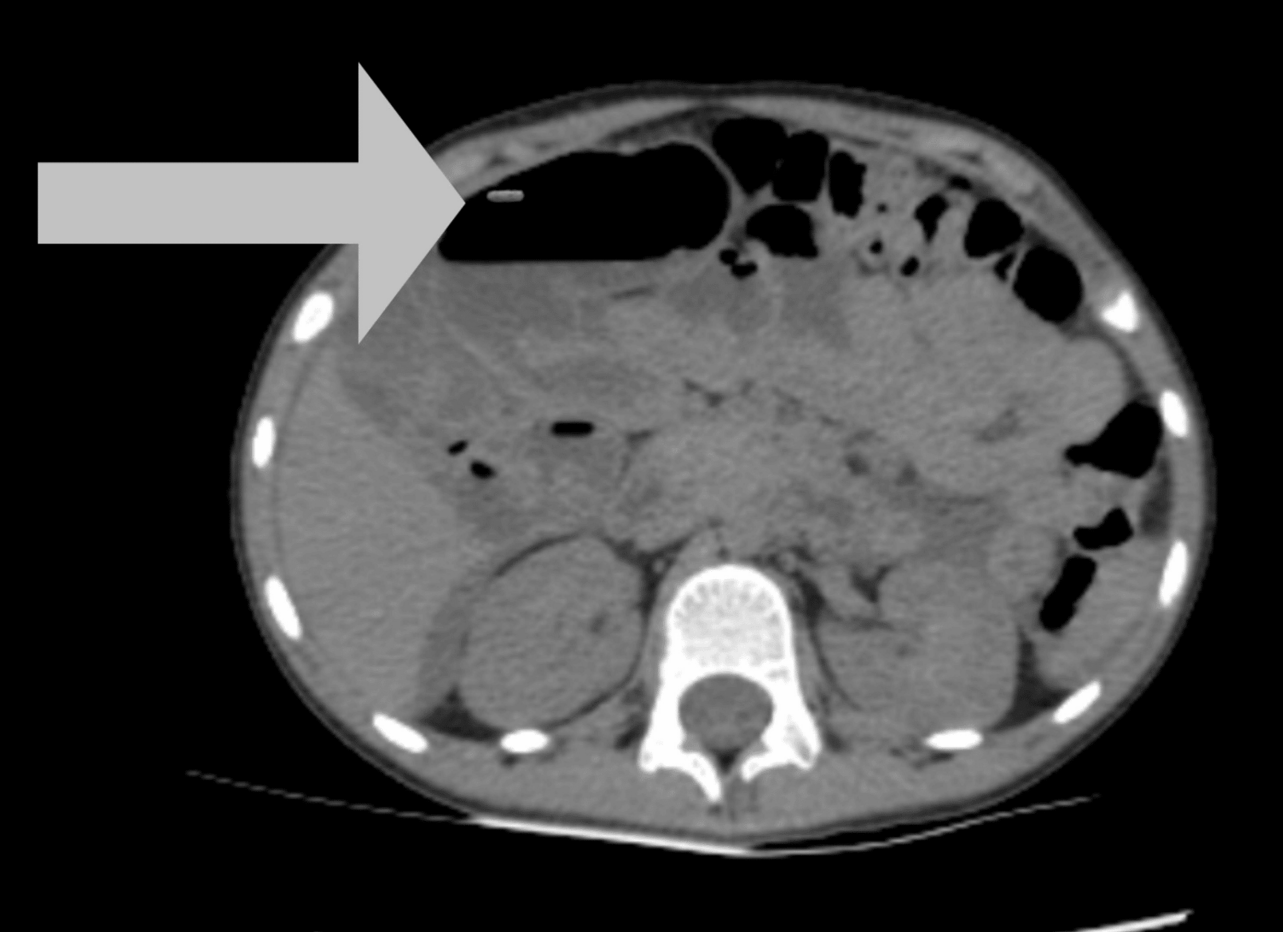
Abdominal computed tomography showing generalized colonic dilation with mural thickening of the sigmoid (arrow) and free peritoneal fluid, suggestive of intestinal obstruction.
